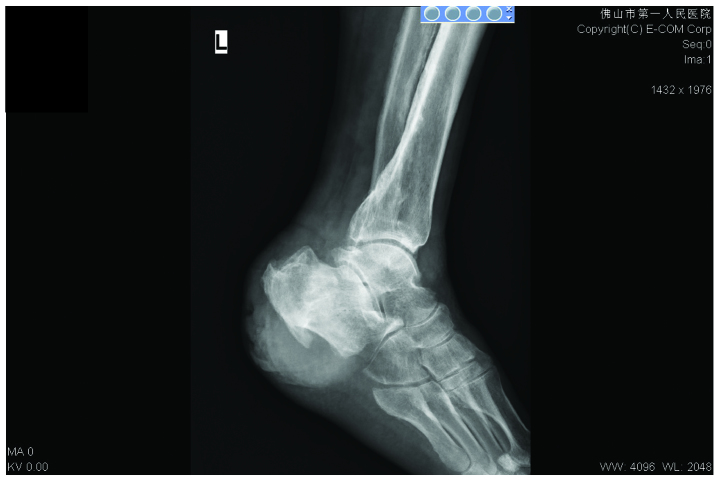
Radiographic findings from the patient shown in Fig. 3, showing bone changes and signs of osteomyelitis.
